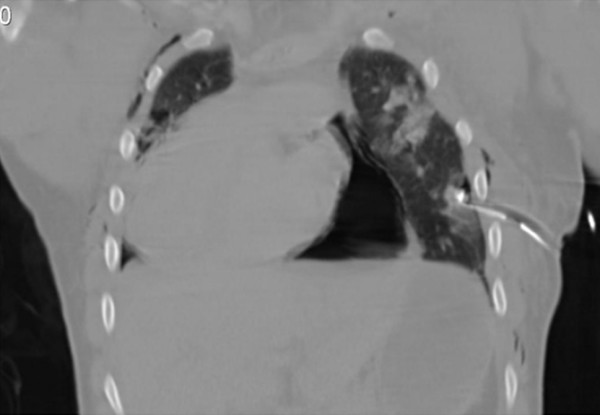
Coronal chest CT demonstrating most of the axial findings including the prominent pneumopericardium and displacement of the heart into the right hemithorax.
